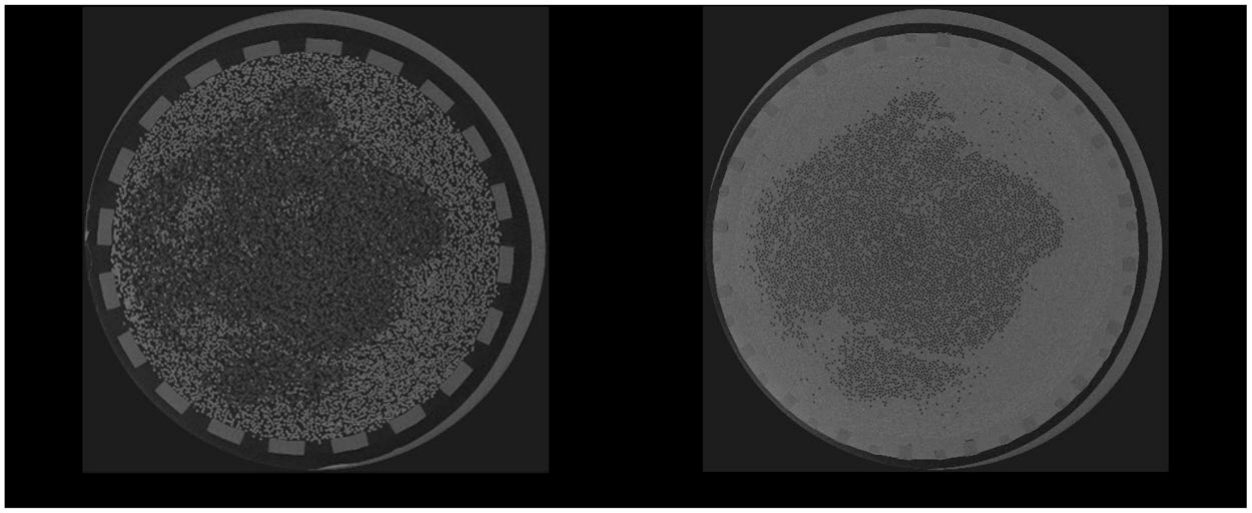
CT images of cross-sections in the same used dialyzer halfway the dialyzer (left panel) and at the central cross-section in the outlet potting (right panel). Central fibers are open and peripheral fibers are blocked. Sections in the potting offer optimal visualization of air inflated open fibers, appearing as black dots.
